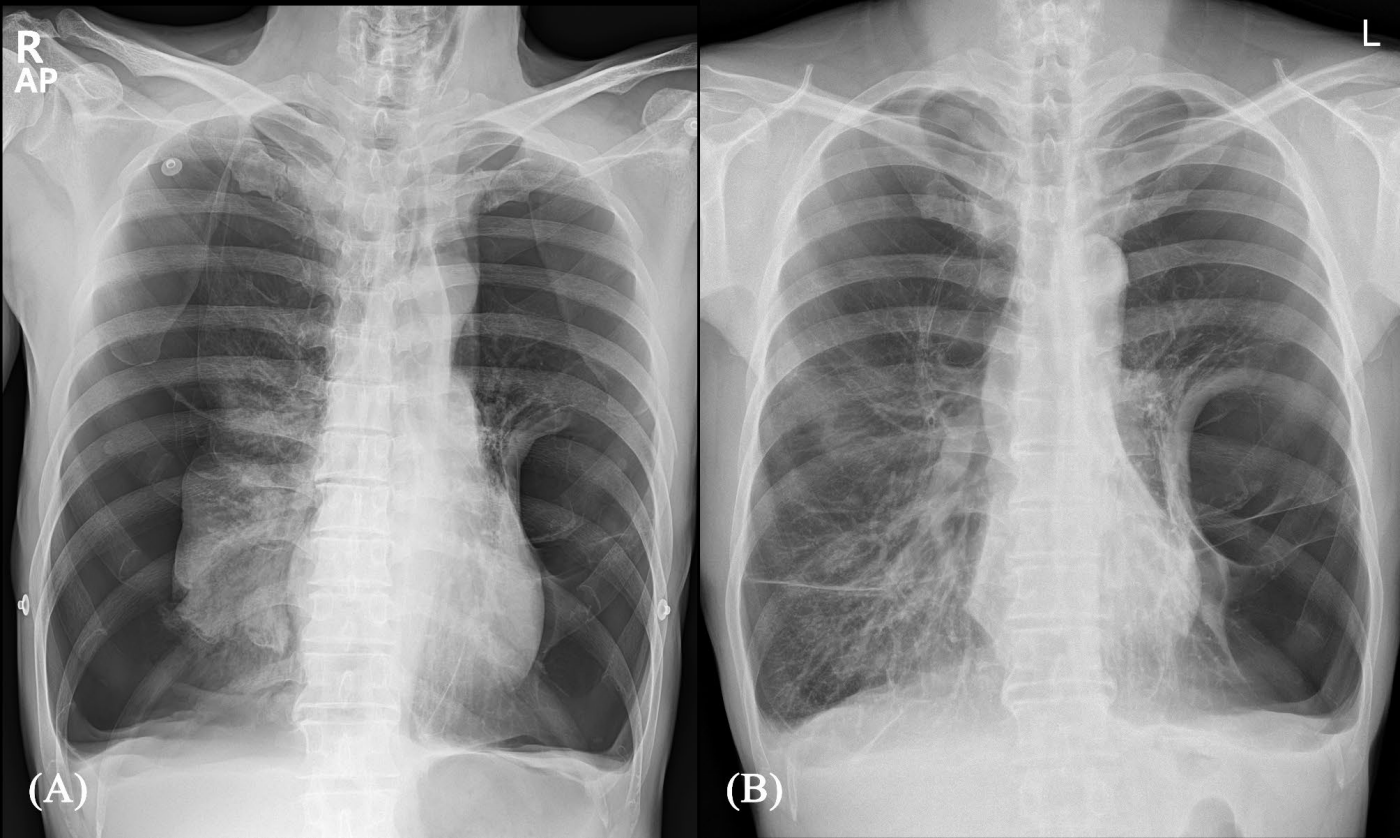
(A) Chest X-ray taken upon the patient’s arrival at the emergency room showing tension pneumothorax on the right side. (B) The most recent outpatient clinic chest X-ray before the development of pneumothorax revealed bullous emphysema
